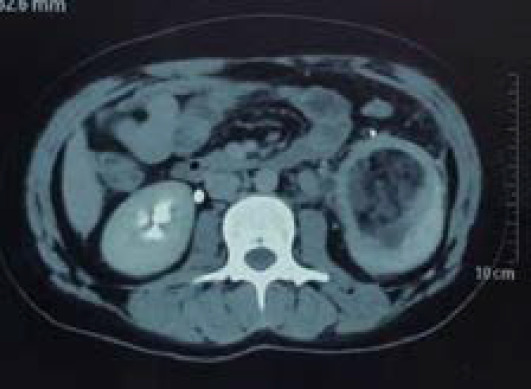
CT image showing cystic lesion in left kidney with internal septations.
